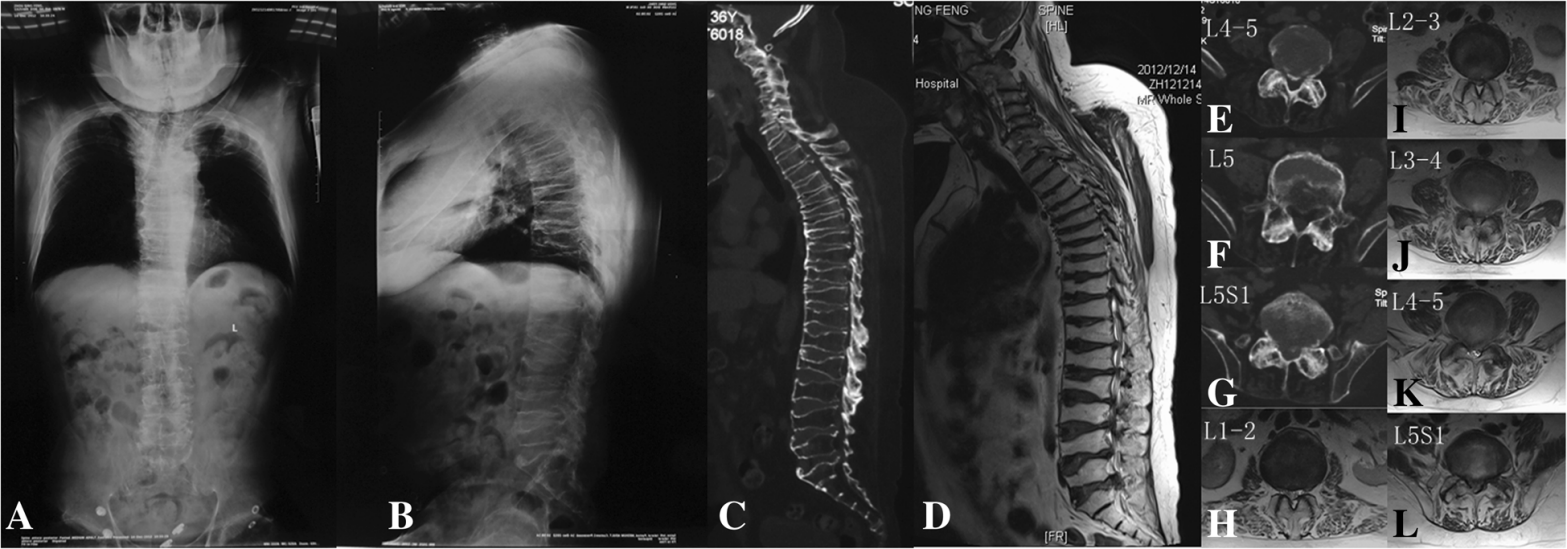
X-ray and CT scan of the whole spine showed osteoporotic flat vertebral bodies and bone bridges at multiple levels (a–c). Axial CT scan revealed maldevelopment of the left pedicle of L5 (f). Ossified herniated discs at L4–5 and L5–S1 were showed (c, e, g). Magnetic resonance imaging showed extensively canal stenosis from L2 to S1 (d, h–l)
